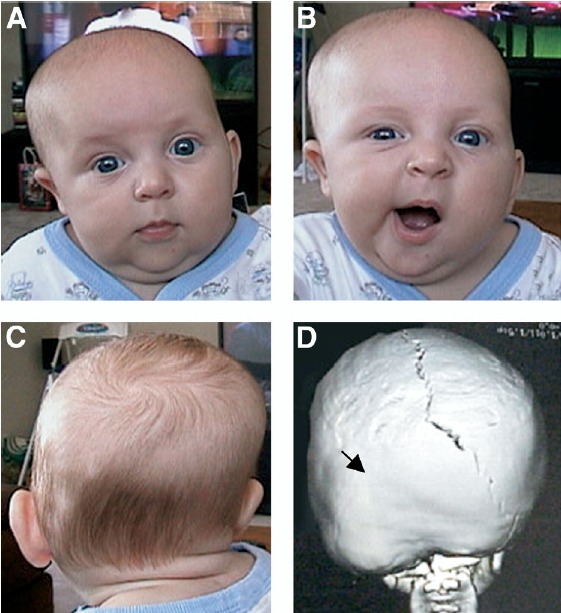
Posterior plagiocephaly caused by lambdoidal synostosis. (A and B) Frontal and (C) posterior views of an infant with left lambdoidal synostosis; the 3D-CT reconstruction (D, posterior view) shows premature fusion of the left lambdoidal suture (arrow). Note the facial asymmetry of the patient secondary to the lambdoidal syostosis (panel B) that does not occur in positional plagiocephaly. Reproducted with permission from: Kimonis Virginia, Gold JA, Hoffman T, Panchal J, Boyadjiev S: Genetics of Craniosynostosis, *Semin Pediatr Neurol*
